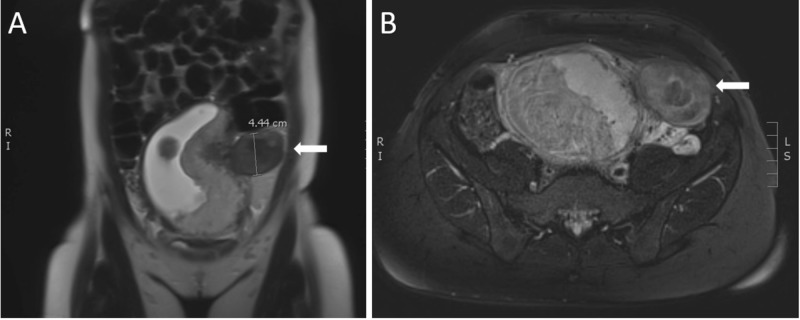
MRI revealing large left exophytic mass with internal hemorrhage (A) T1-weighted coronal image with height measurement depicting mass (white arrow). (B) T2-weighted axial image depicting the same mass (white arrow).
